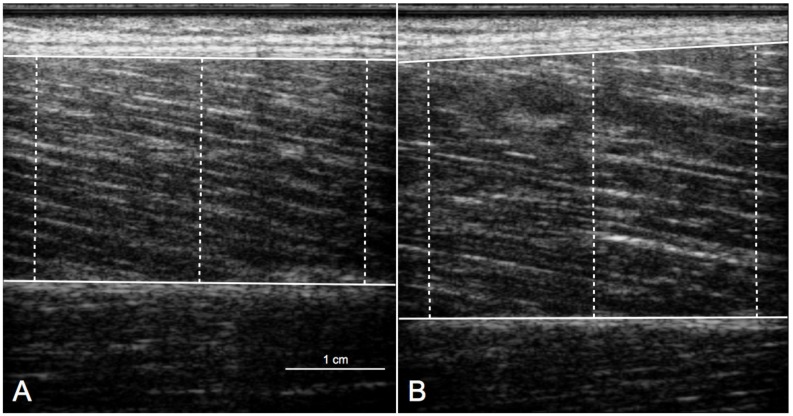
Representative recordings of longitudinal ultrasonographic images of the vastus lateralis muscle on the involved (A) and uninvolved side (B). The horizontal solid lines represent the superficial (upper line) and deep (lower line) aponeuroses. The vertical dotted lines represent the muscle thickness measured at three different spots (left, middle, right). This patient had anterior cruciate ligament reconstruction with meniscal surgery and showed a −15% side-to-side asymmetry in vastus lateralis thickness.
